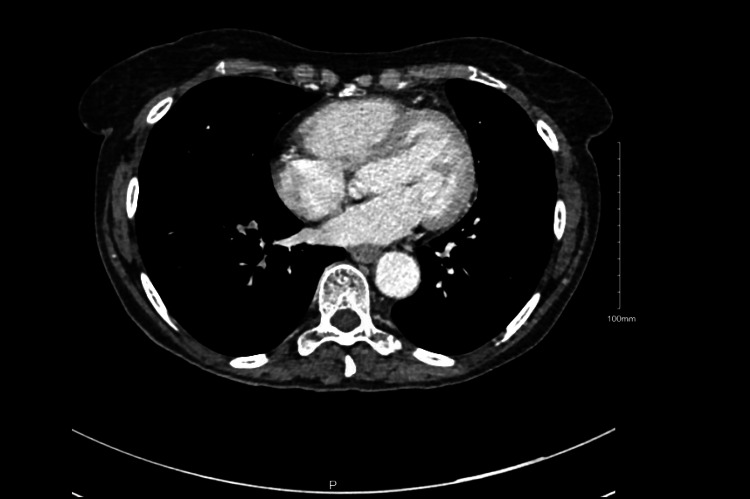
CT scan CT scan from admission with a complete radiographic response of the RV mass following radiotherapy. RV: right ventricle
